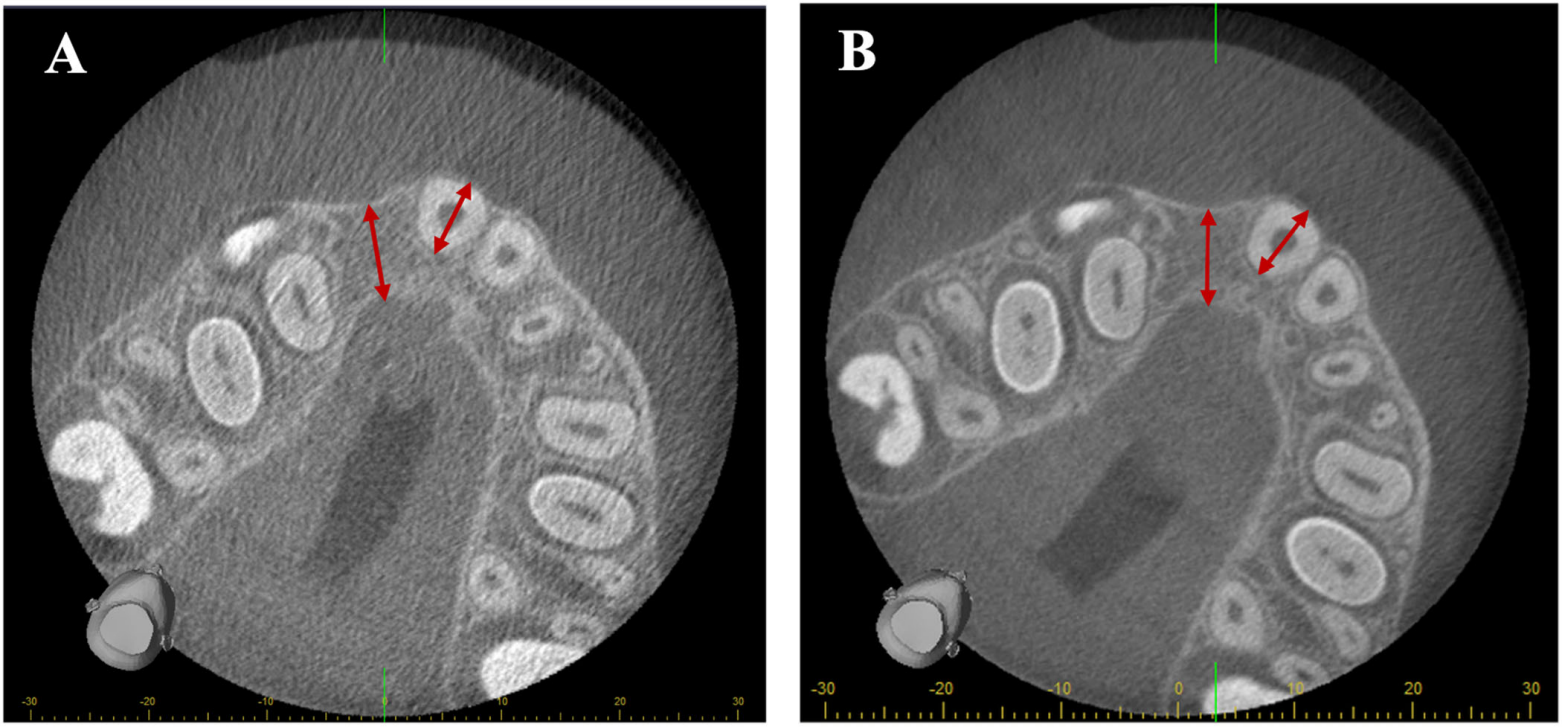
CBCT examinations showing the comparison of graft thickness with the root width of the tooth adjacent to the cleft following alveolar bone grafting made with (A) a low‐dose (2 mA) protocol and (B) a standard‐dose protocol (5 mA).
